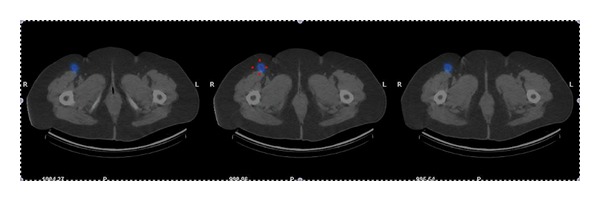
73-year-old woman with melanoma in the right leg (Breslow 3.8 mm) diagnosed in January 2011. It was resected in March of that year. PET/CT was performed in April 2011 with negative results. SLNB was undertaken in May 2011 (SPECT-CT images in axial view) showing a SN in right groin. Excision was performed with SN pathology negative for malignancy.
